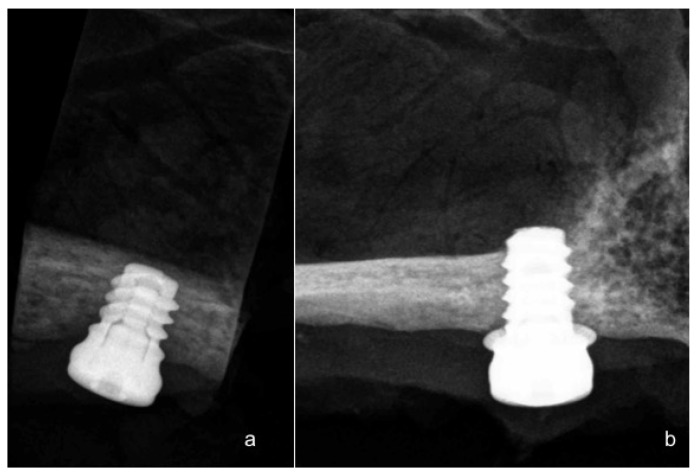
(a) Narrow ring extra-short implant radiograph after 90 days of evaluation; (b) Wide ring extra-short implant radiograph after 90 days follow-up.
